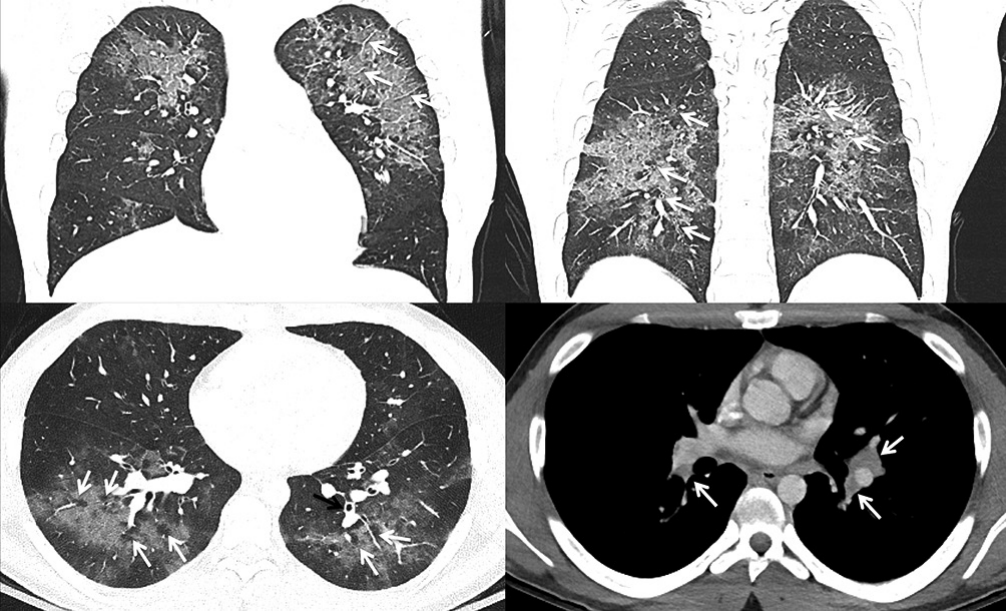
A (top left). Coronal CT image showing bilateral upper lobe peribronchovascular groundglass opacities, with mild septal thickening in the left upper lobe (white arrows) B (top right). Coronal CT image showing bilateral lower lobe peribronchovascular groundglass opacities, with bronchial wall thickening (white arrows) C (bottom left). Axial HRCT image showing bilateral lower lobe peribronchovascular groundglass opacities with unusual small areas of sparing in the immediate peribronchovascular space (white arrows) and bronchial wall thickening (black arrow) D (bottom right). Axial CT image showing mild bilateral hilar lymphadenopathy (white arrows). HRCT, high-resolution CT.
